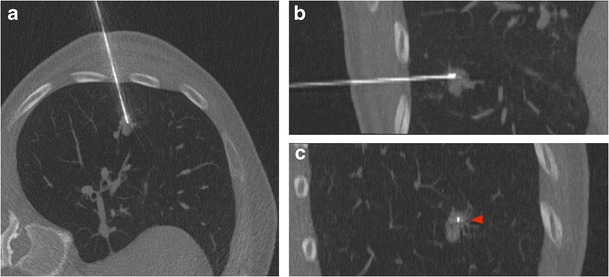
CT-guided chest biopsy of a pulmonary nodule in the left lower lobe. (a) Axial, (b) coronal and (c) sagittal intraprocedural CT scans showing the needle tip in the lesion (arrow)
